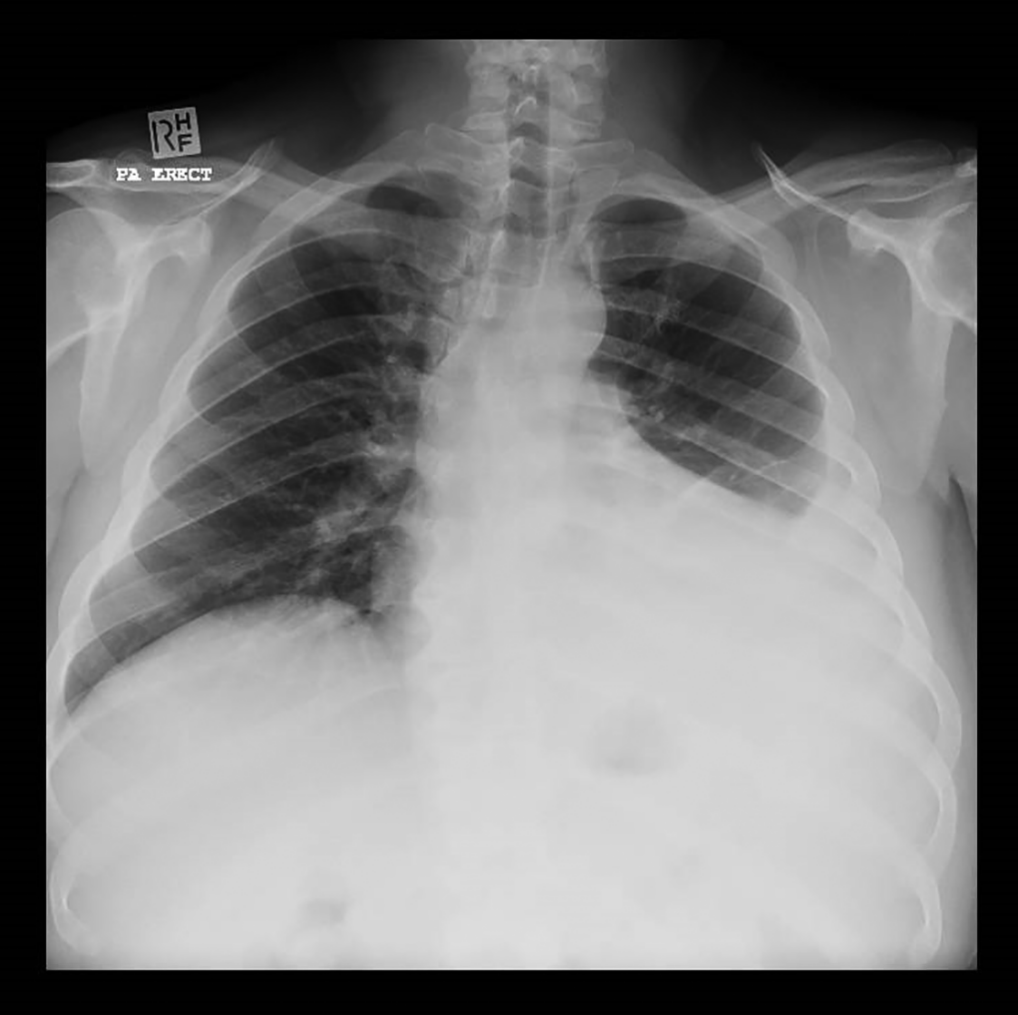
Plain chest radiograph demonstrating a unilateral left‐sided pleural effusion.
